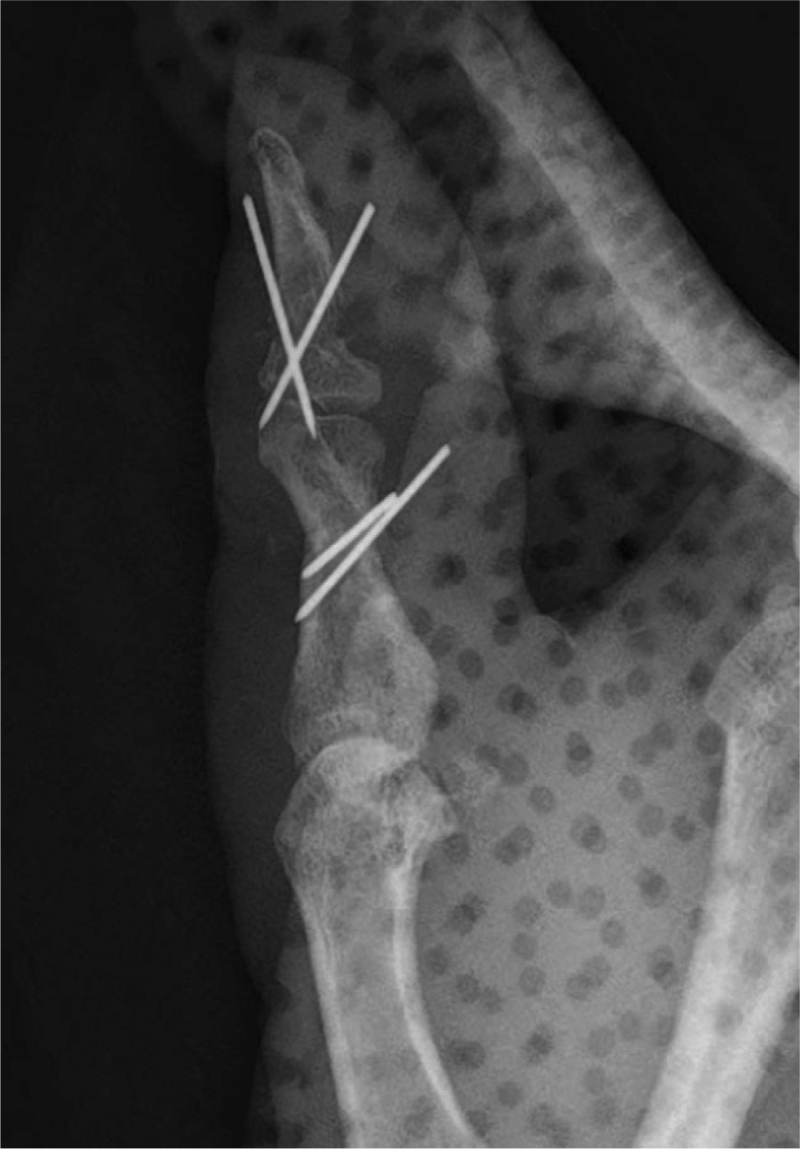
Postoperative x-ray of the affected finger.
